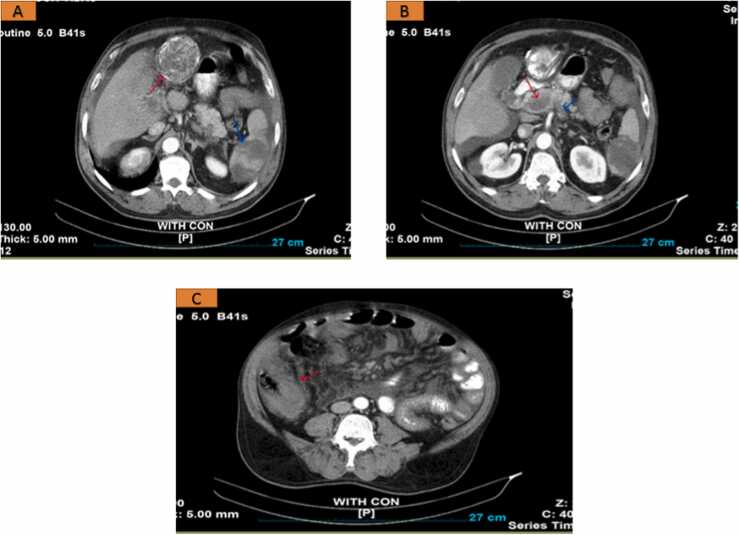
An abdominal CT scan with oral and IV contrast on a patient with portal, splenic and mesenteric venous thrombosis associated with HCD.
